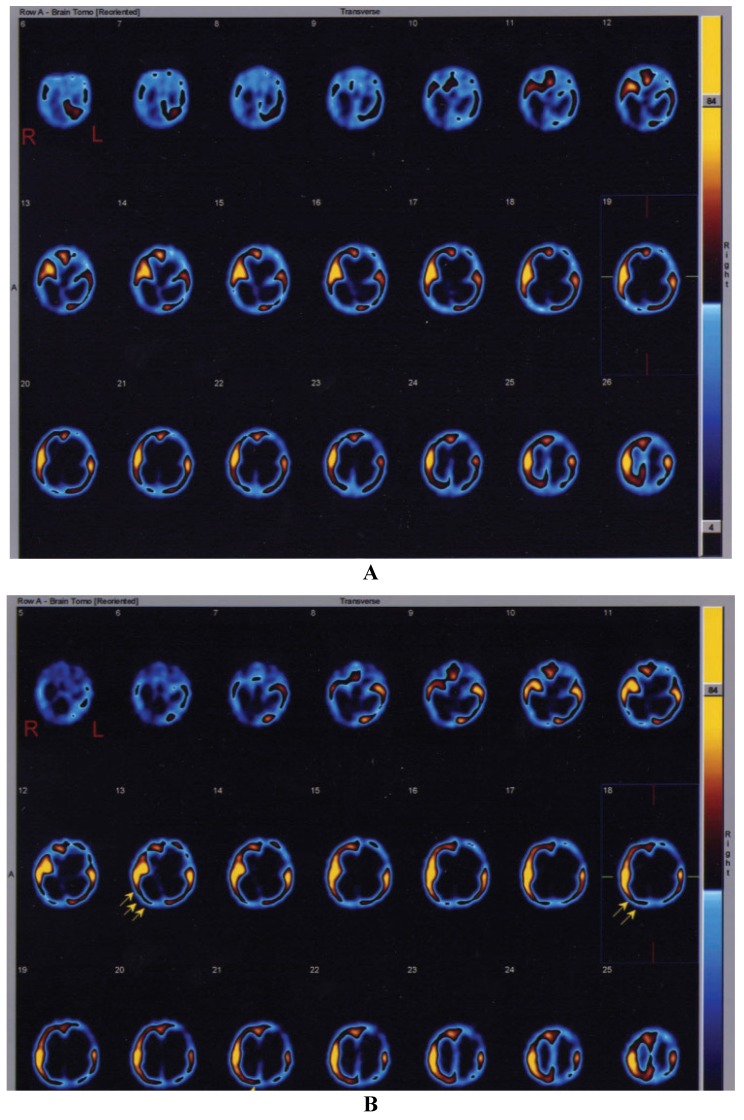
Single-photon emission computed tomography (SPECT) study showing the brain perfusion pattern in the second infant affected by severe hypoxic-ischemic injury. (A) Before treatment. (B) After intraventricular NGF infusion. The arrows in (B) highlight the sites of improvement of cerebral perfusion in right temporal and occipital cortices.
